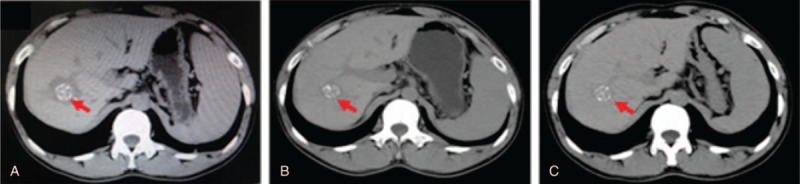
The computed tomography (CT) scan of the upper abdomen for the HAE patients at 1, 6, 12 months after the microwave ablation treatment. A, CT scan 1 month after surgery. B, CT scan 6 months after surgery. C, CT scan 12 months after surgery. The red arrow indicates the HAE lesion. HAE = hepatic alveolar echinococcosis.
